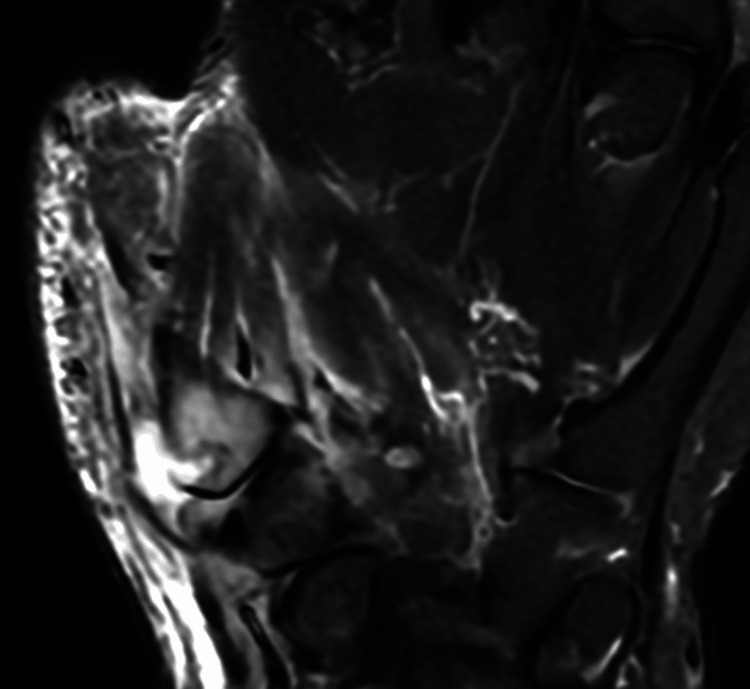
Magnetic resonance imaging T2-weighted magnetic resonance image of the right hand in Case 2 showing inflammatory changes extending into the bone marrow of the first metacarpal bone
